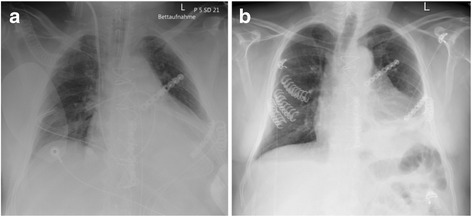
Chest X-ray showing chest wall reconstruction at the time of initial surgery (a) and 3 days postoperatively (b)
